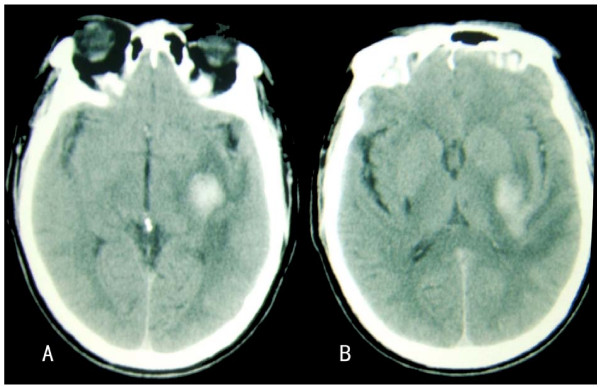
(A) *CT scan at a level corresponding to the *mid-thalamus, showing the hemorrhage located laterally to the posterior limb of the internal capsule. (B) *CT scan with hemorrhage at the level of the left *putamen, its posterior aspect is adjacent to the posterior part of the posterior limb of the internal capsule, and the posterior horn of the lateral ventricle compressed.
